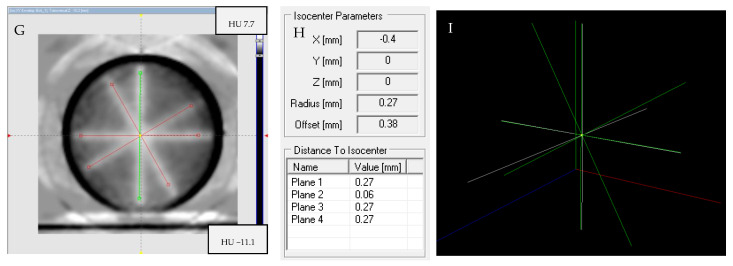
Isocenter determination. Processing of iCBCT images of PABIG^nx^ in PH-5-DD1 GeVero Co. container irradiated with the TrueBeam accelerator (mode: Pelvis): (A) is for the mean series (calculated from three series) with the mean background (calculated also from three series) subtracted; (B) corresponds to the isocenter results of (A), and (C) is for the 3D visualization of the isocenter determined in (A); (D) is for the mean series (calculated from three series) filtered with the Envelope filter (mean, 3 iterations, Kernel = 3, Kernel mode = 2D, Kernel unit = pixels) with the mean background subtracted (calculated from three series); (E) corresponds to the isocenter results, and (F) is for the 3D visualization of the isocenter determined in (D); (G) is like (D), however, the background was also filtered with the Envelope filter (same parameters); (H) is for the isocenter results for (G), and (I) is the 3D visualization of the isocenter determined in (G). The colored lines in (A,D,G) intersect at the isocenter. The isocenter is marked with a yellow dot.
